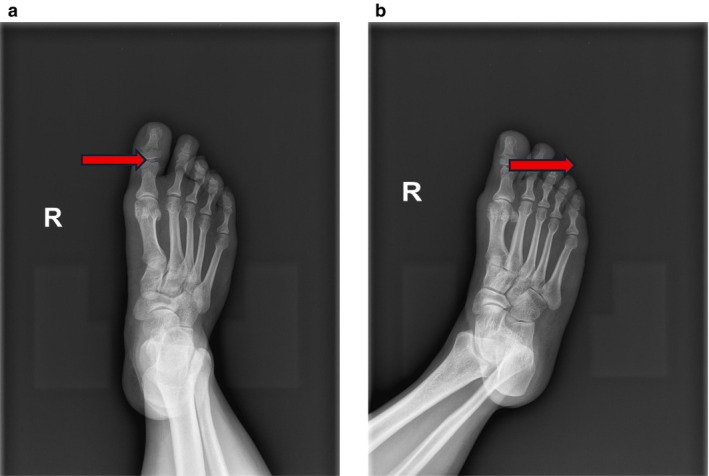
Preoperative radiograph of the right foot reveals a clear fracture of the distal phalanx and the presence of an intra‐articular sesamoid bone within the interphalangeal joint. The structure indicated by the red arrow corresponds to the sesamoid bone.
